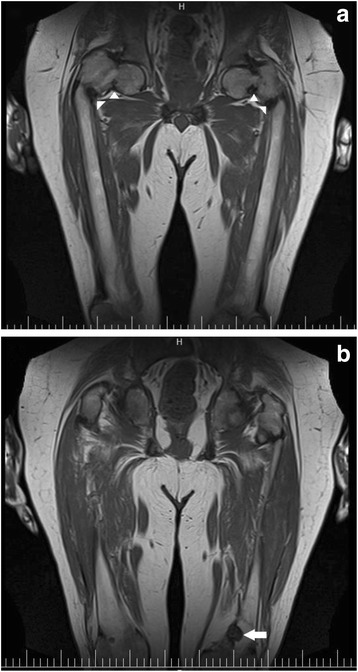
T1 weighted MRI: a Femoral neck and subtrochanteric fractures on both sides (arrow heads), and bilateral coxa vara deformities; b A mass-like lesion in medial aspect of the left distal femur metaphysis (arrow)
